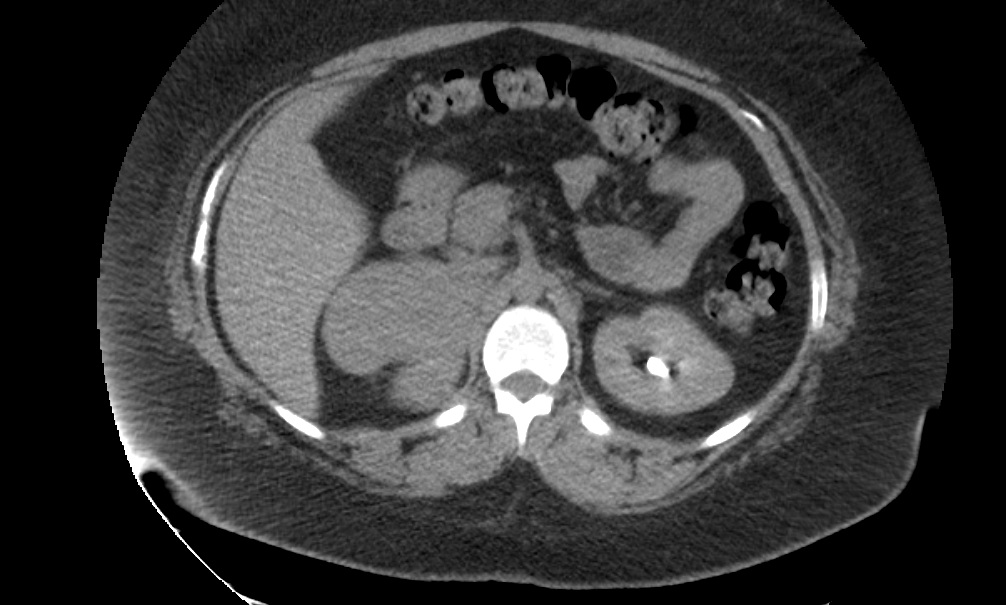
Preoperative CT of the abdomen and pelvis (axial view) showing multilobulated mass involving the right adrenal gland.
